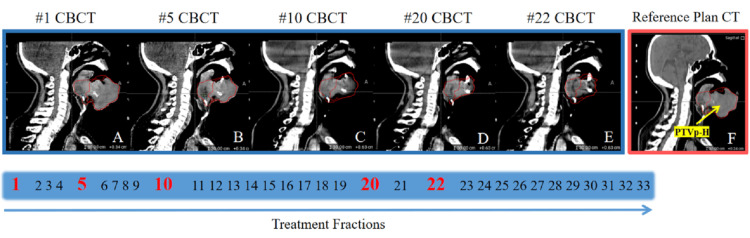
Target changes in different treatment fractions of OART in the sagittal plane Figures A to E are the sagittal plane of the same layer of cone beam computed tomography (CBCT) and target in the first to fifth OART, and Figure F is the sagittal plane of the same layer of the Sim CT target. The following is the time axis of 33 treatment fractions, in which the number of online ART fractions is displayed in bold red. OART: online adaptive radiotherapy.
